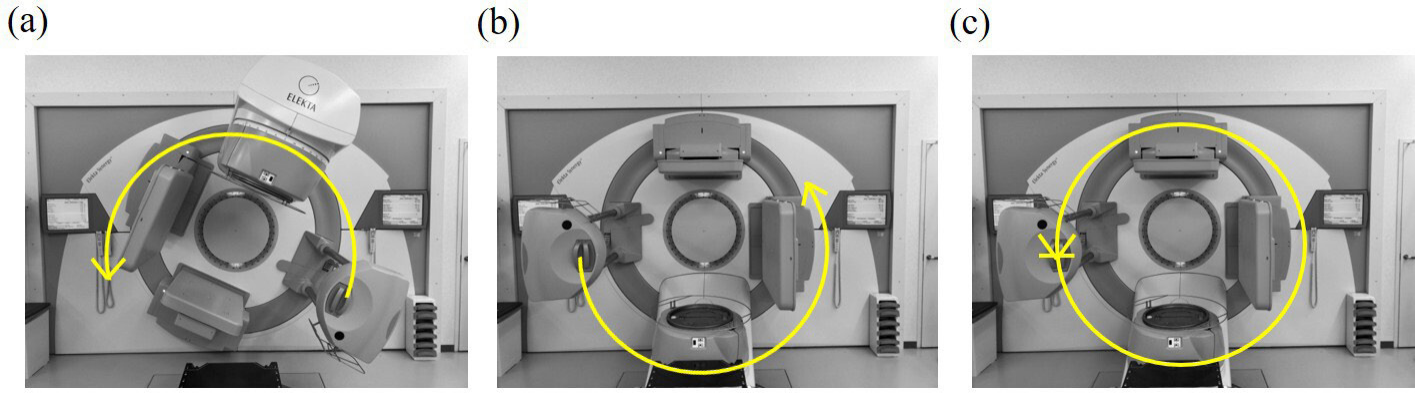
The rotational direction of the X-ray tube in each protocol in Table 2: (a) protocol 1, (b) protocol 2, and (c) protocol 3
